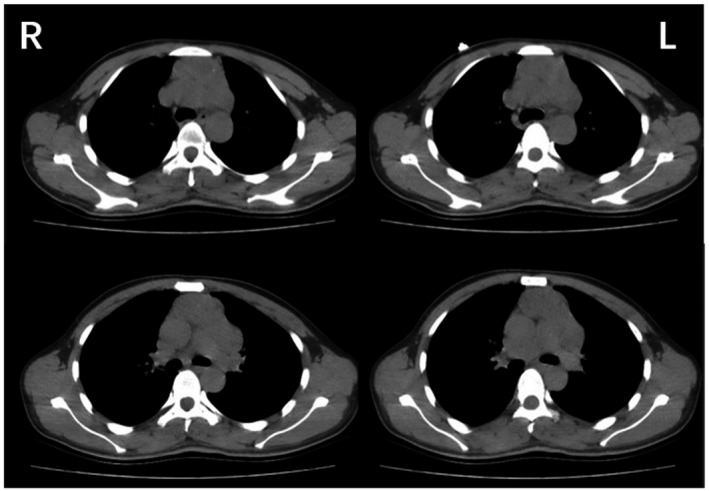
Chest computed tomography (CT) scan showing a mass in the anterosuperior mediastinum. Most of the lesions, which were wavy and fused together, were located in the anterior superior mediastinum
